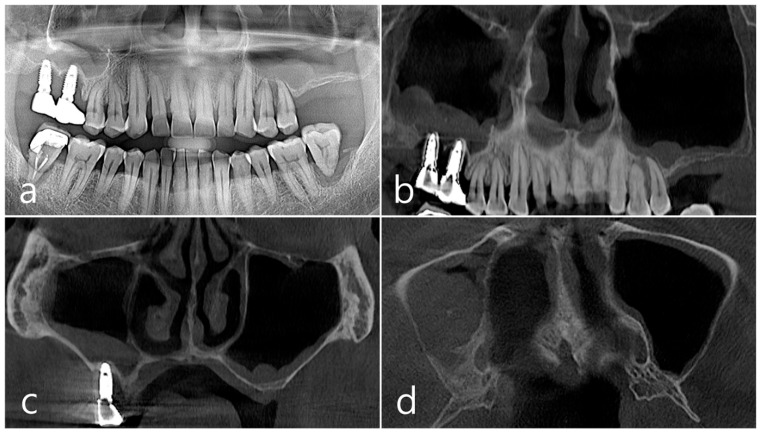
Case 2. (a,b) On the preoperative panoramic radiography and panoramic image of CBCT, the residual alveolar ridge in the posterior maxilla was severely atrophied and pneumatization of maxillary sinus was severe; (c,d) In CBCT imaging, mucosal thickening (arrows) ranging from 2 to 15 mm was observed in the sinus floor, depending on the location, but there was no mucosal thickening in the rest of the sinus wall.
